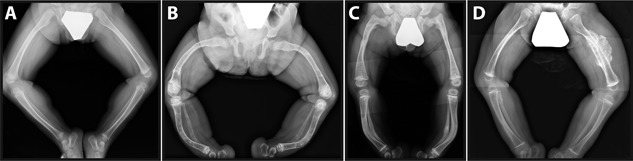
Lower extremity radiographs. (*A*) A 21‐month‐old boy with osteogenesis imperfecta (OI) type I caused by a frameshift mutation in *COL1A1*. (*B*) A 3‐year‐old girl with OI type III caused by a glycine substitution in *COL1A2*. Note the severe bowing of both femurs and tibias, and healing fracture of the distal right femur. (*C*) A 3‐year‐old boy with OI type IV caused by a valine deletion in *COL1A2*. Note the deformities of both femurs and tibias, and healing fracture of the left fibula. (*D*) A 19‐month‐old boy with OI type V caused by a *IFITM5* mutation. Note the hyperplastic callus formation of the left femoral shaft and radiodense metaphyseal bands adjacent to the growth plate.
